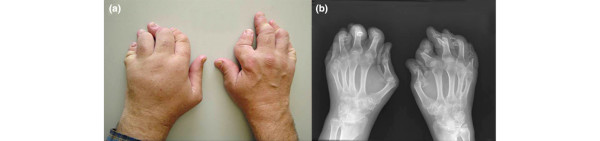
A patient with arthritis mutilans with digital shortening. (a) Clinical photograph. (b) Radiograph of the hands.
